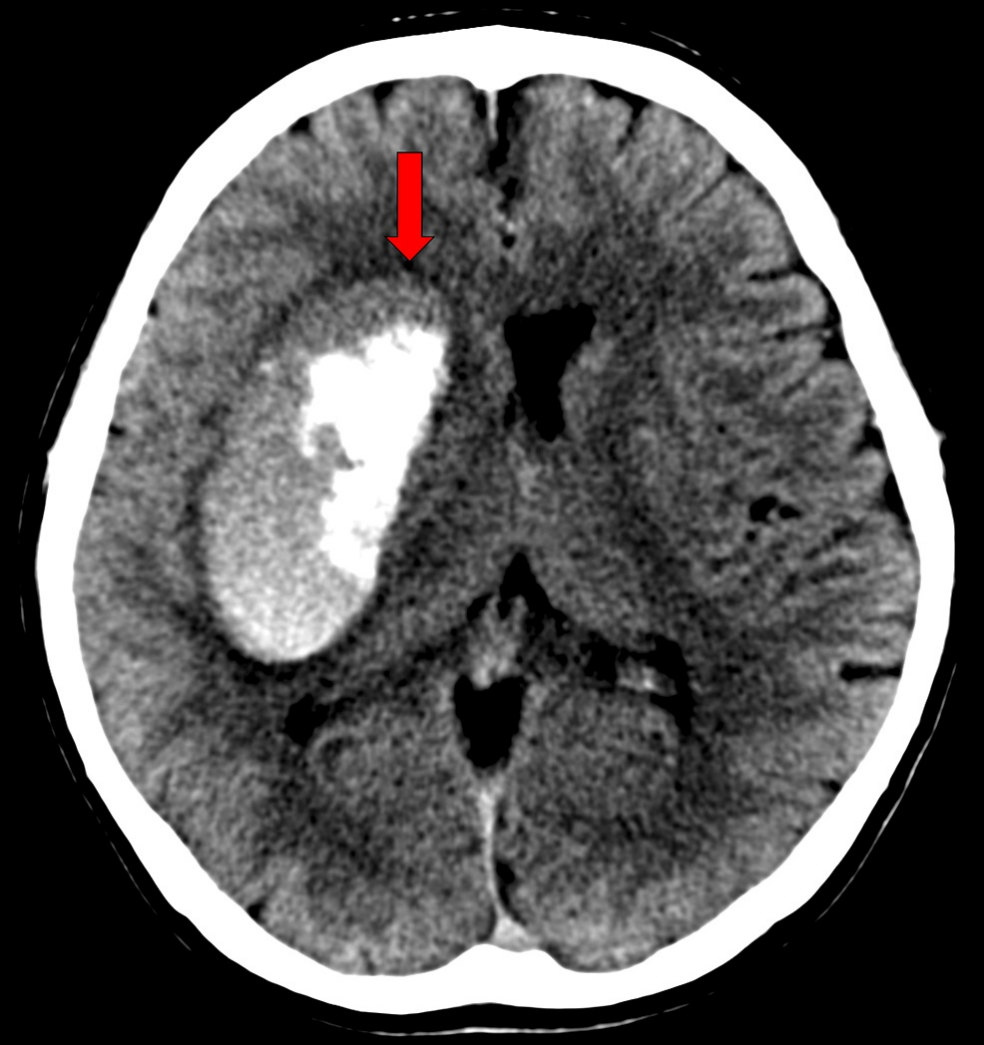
Cranial CT scan showing a right putaminal hemorrhage with compression of the right lateral ventricle and midline shift
